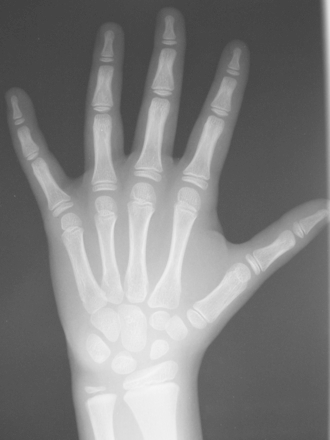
Left-hand/wrist radiograph.
